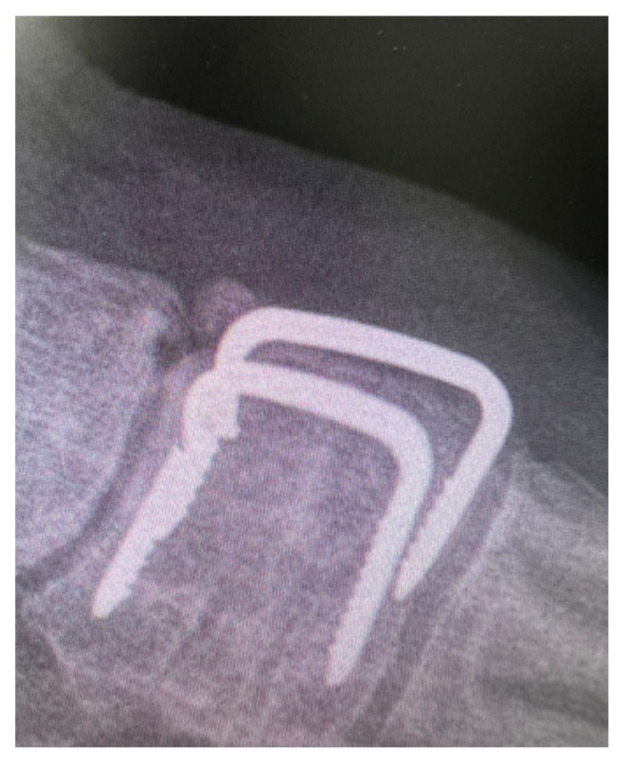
Lateral midfoot radiograph depicting broken metalwork 6 months following tarsometatarsal joint fusion.
